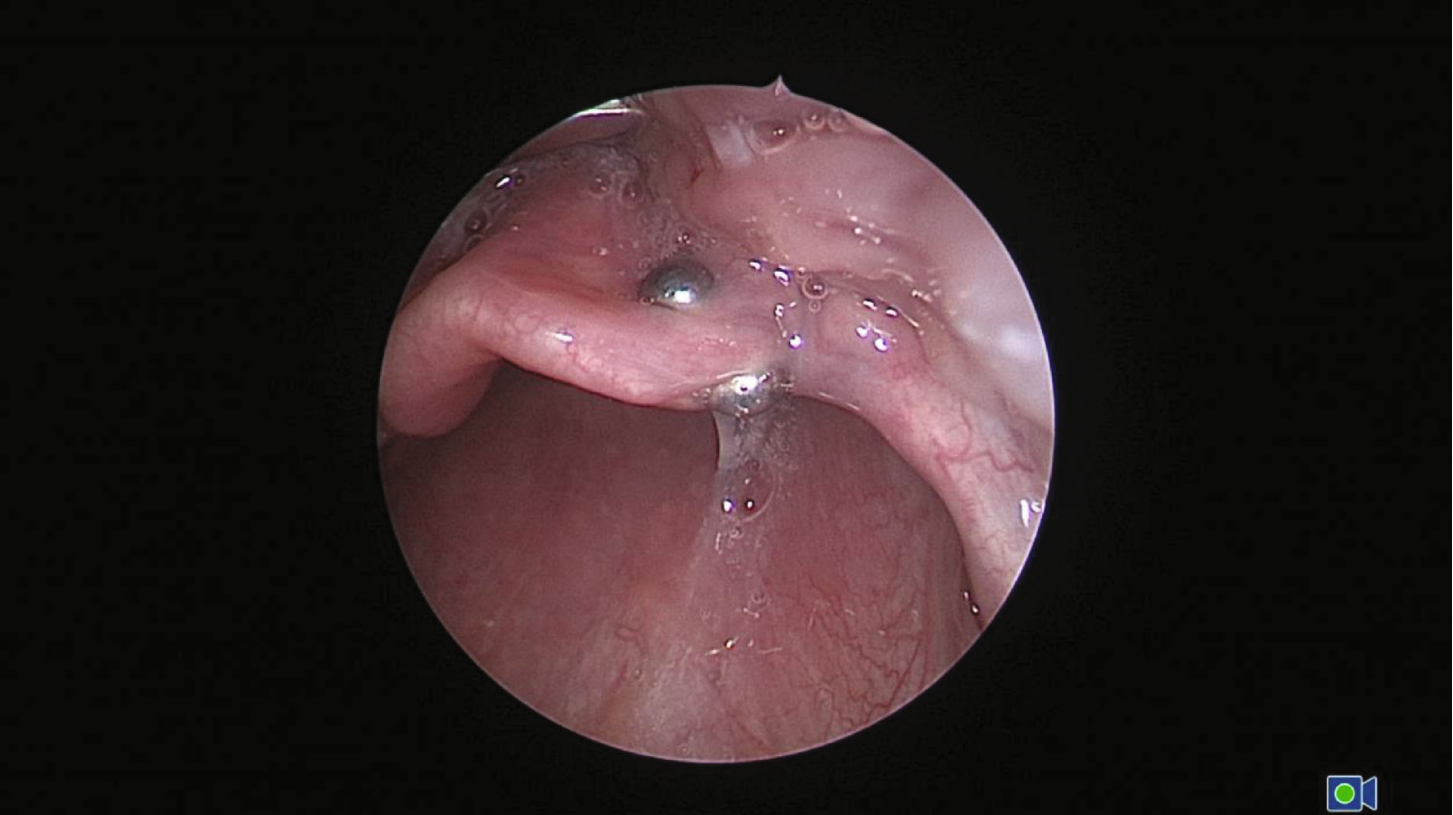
Intraoperative image demonstrates ball magnets along the ventral and dorsal side of the epiglottis, corresponding to radiographic findings.
